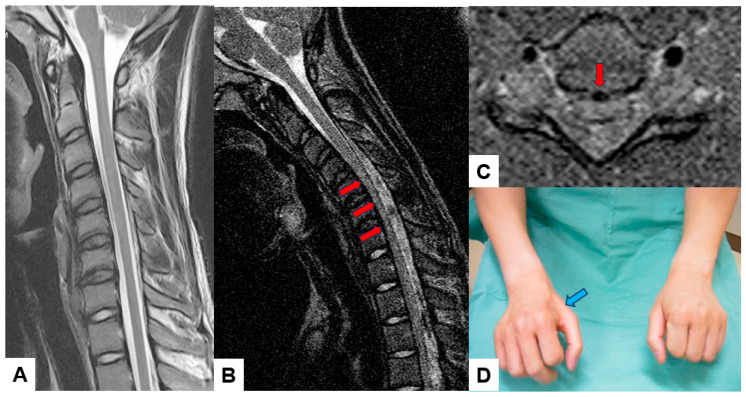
Sixteen-year-old male, Hirayama disease, (A) cervical midsagittal T2-weighted MRI, (B) cervical midsagittal MRI in flexion position, (C) cervical axial T2-weighted MRI at C5/6, (D) bilateral hands of the patients. Red arrows indicate spinal cord compression in the flexion position. A blue arrow shows muscle atrophy in his right hand.
